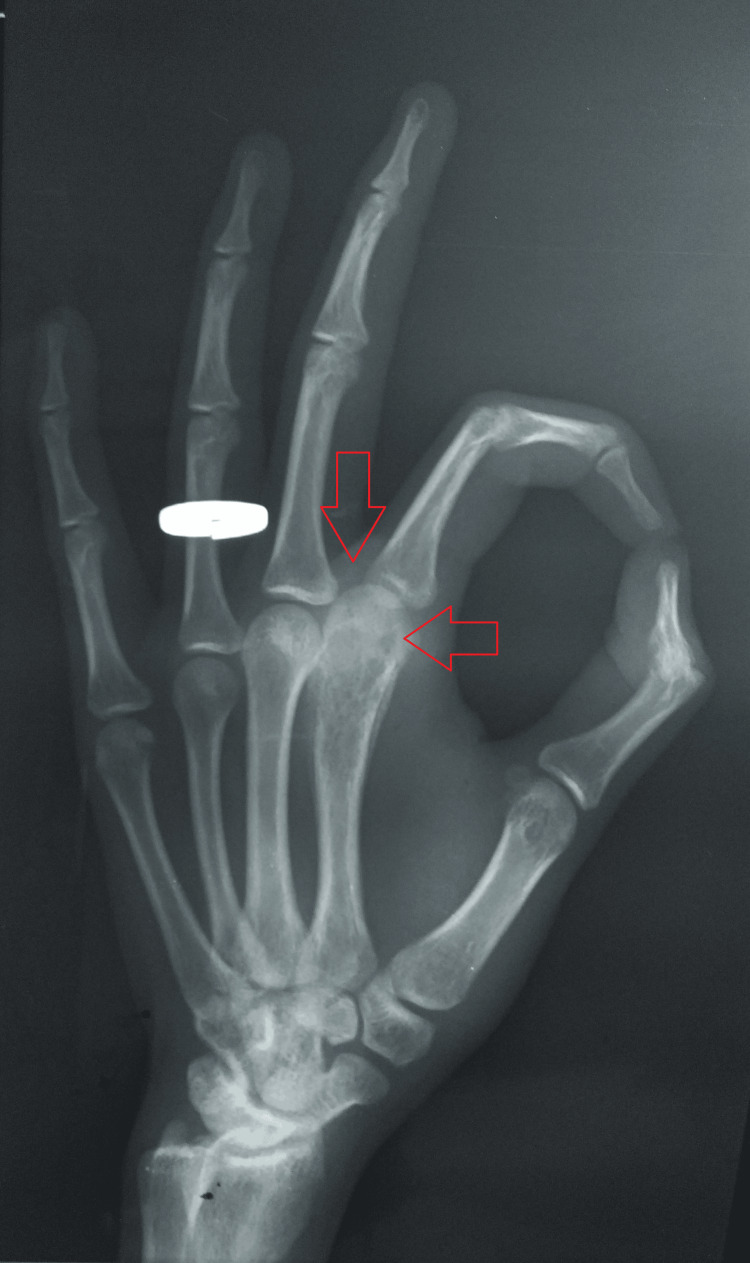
A plain radiograph-oblique view of the hand showing a lytic lesion with slight cortical erosion and minimal periosteal reaction over the head of the second metacarpal of the left index finger
